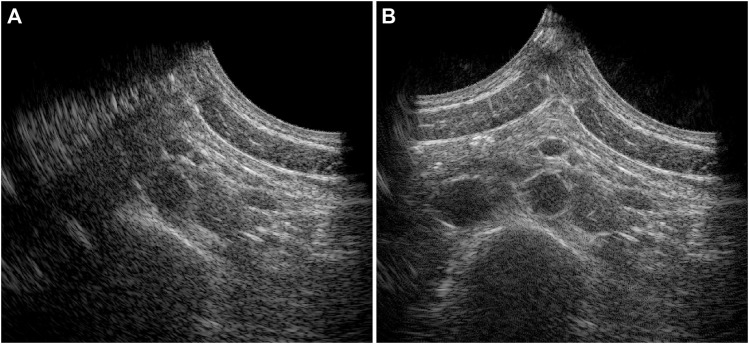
Full field-of-view images of the single-perspective (A) and bistatic dual-aperture ultrasound acquisitions (B) of the abdominal aorta in volunteer 1.
